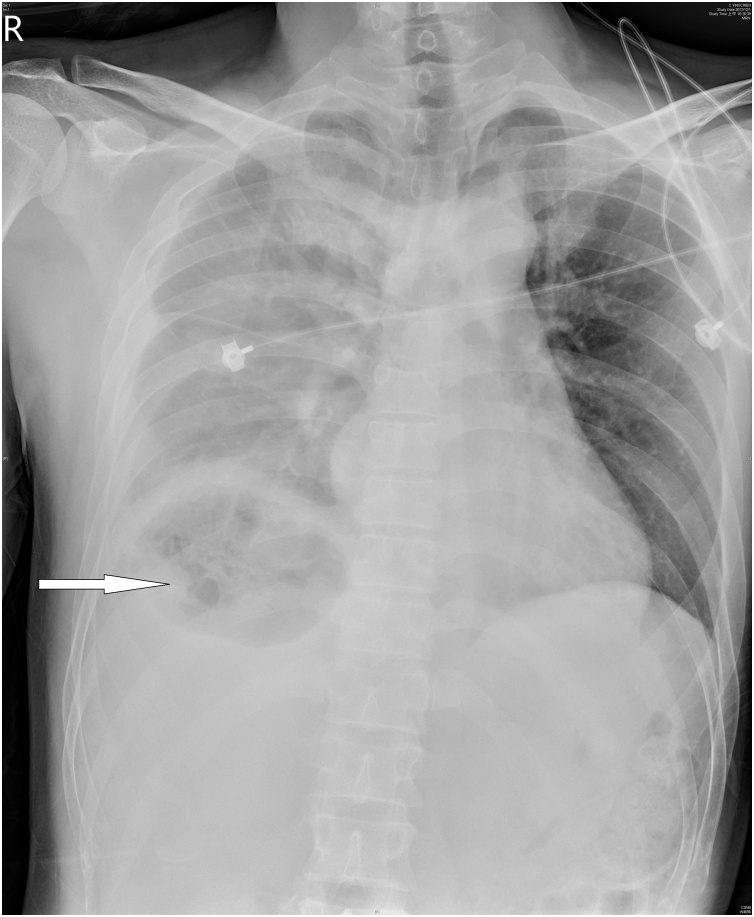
Chest radiography of the same patient performed 58 h after first abdominal computed tomography scan, showing right subphrenic abnormal entrapped air (arrow).
